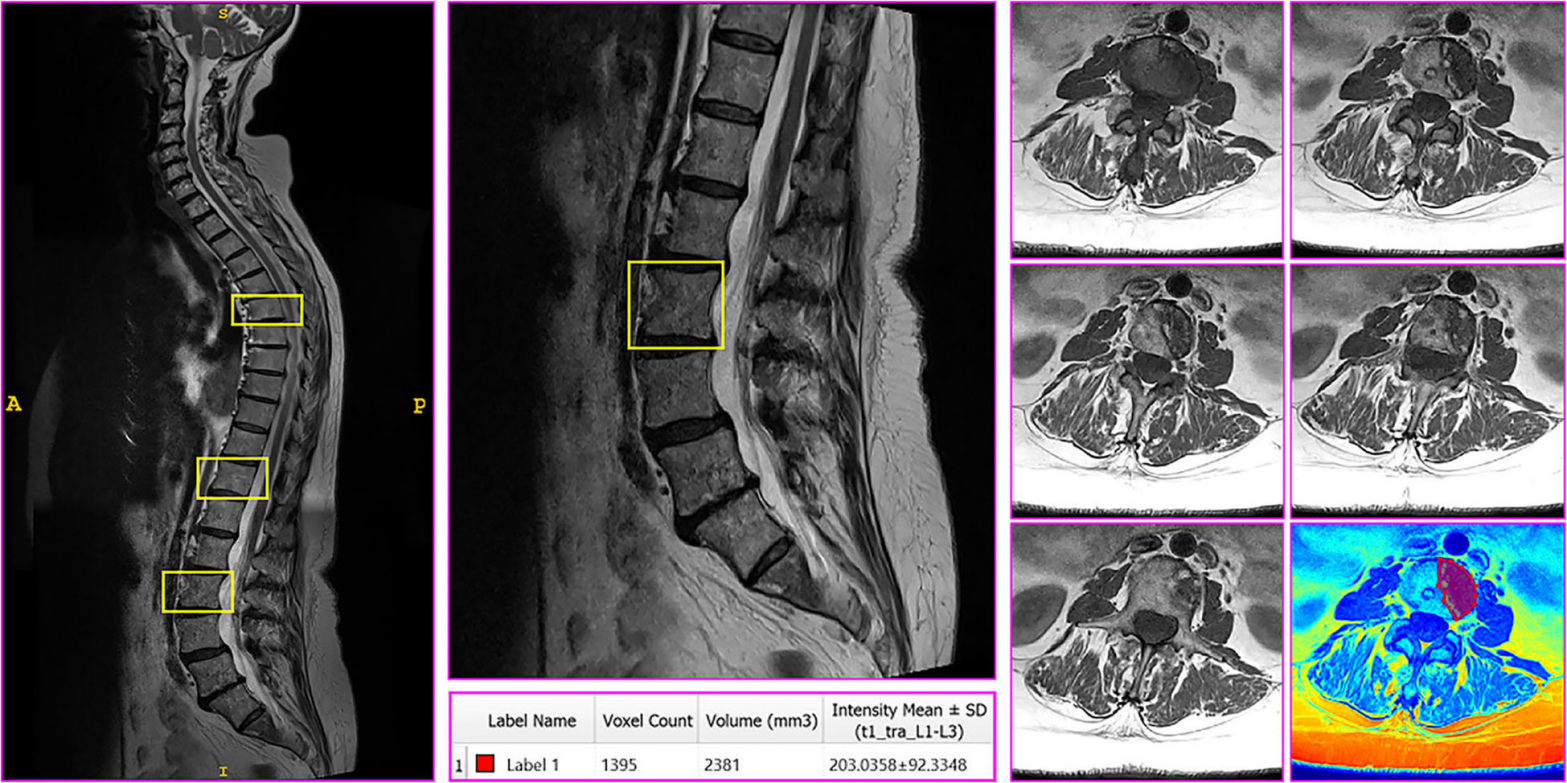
Example of magnetic resonance imaging (MRI) image acquisition. Left: cervical, thoracic and lumbar scout views stitched together with three osteolytic lesions identified at T5, T11 and L2. Top, middle: lumbar scout view at higher resolution with the L2 vertebra outlined. Bottom, middle: example data outputs provided by regional analysis. Right: slice by slice, cephalad to caudal, transverse view at each level of the L2 lesion, with an example colour map and tumoural analysis of one slice in isolation
